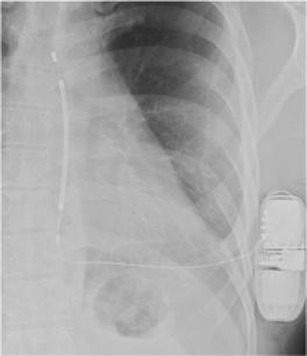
The subcutaneous electrode is implanted in the left parasternal position. There is currently only one manufacturer with this device (Cameroon Health/Boston Scientific)
